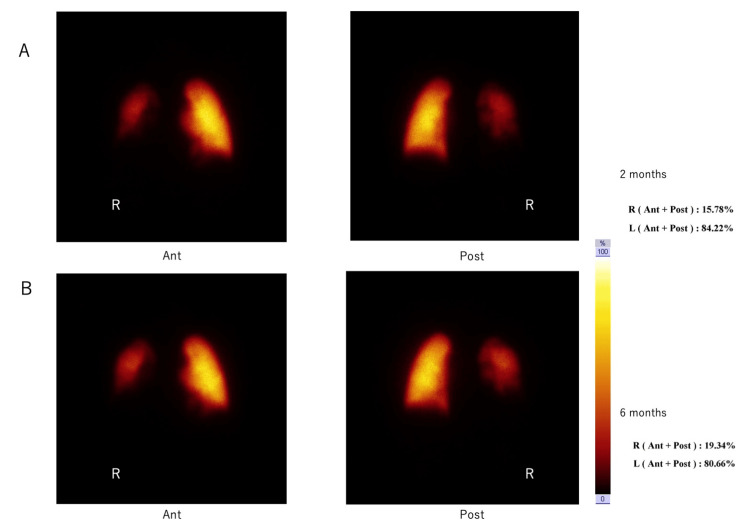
Pulmonary blood flow ratios between the autologous and transplanted lungs in Case 1, at different times post-surgery: pulmonary perfusion scintigraphy (PPS) results. A: 2 months, B: 6 months, R: right, Ant: Image taken from the front, Post: Image taken from the back The number indicates the percentage, which is 100% including the left and right sides.
